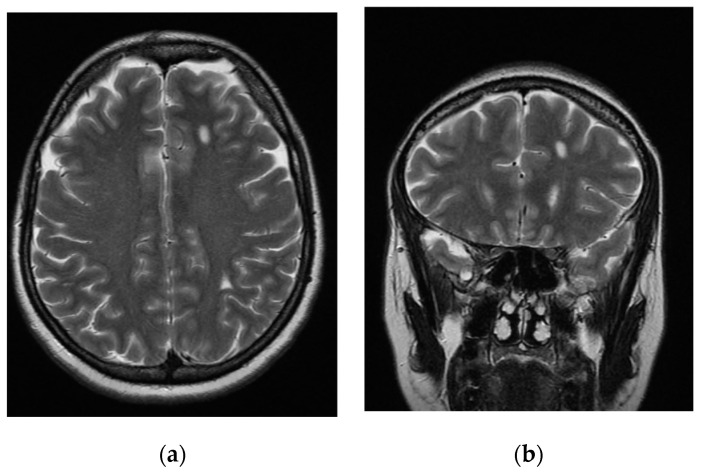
Axial (a) and coronal (b) fast-spin echo T2-weighted magnetic resonance imaging (MRI) showing two focal gliotic lesions in the subcortical white matter of the left hemisphere in the anterior frontal region (a,b) and in the postero-inferior parietal region (a).
